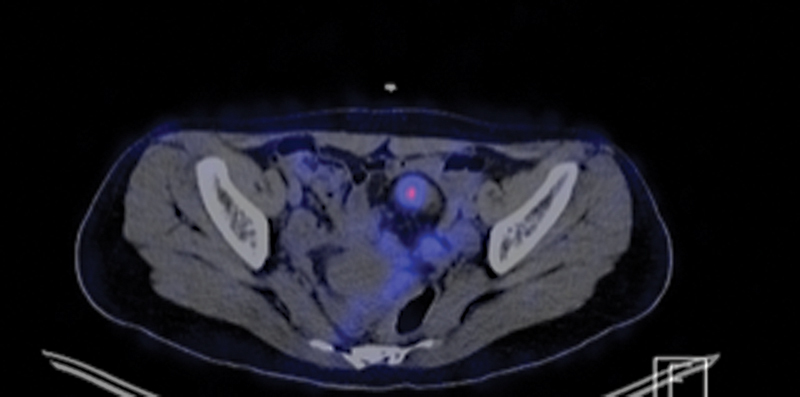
SPECT-CT images of a 15 year old adolescent girl with verified Ectopic Gastric Mocase at a Dublication cyst as determined by Meckel's Diverticulum Scintigraphy.
